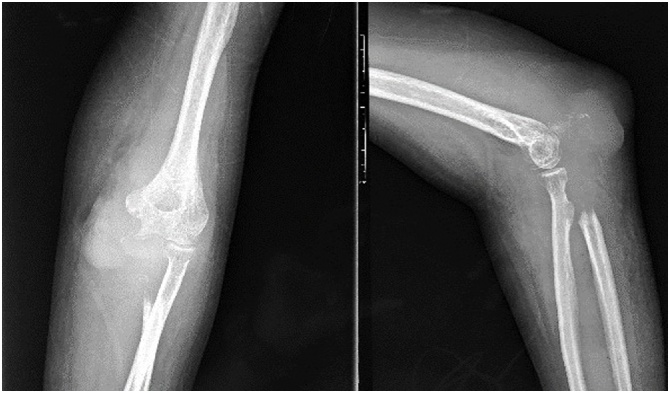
Pre operative x-ray of left elbow. There is lytic area in proximal of the ulnar bone.
